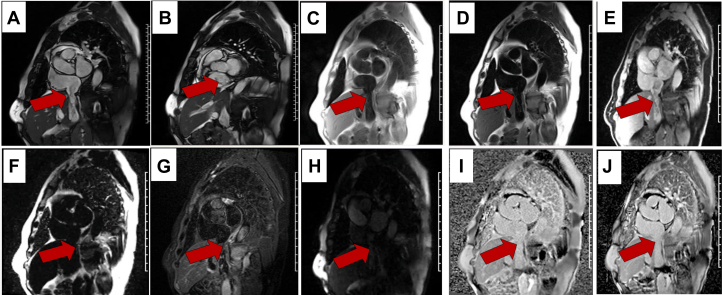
Cardiac MRI Findings Cardiac MRI demonstrating a lobulated, spongy intraluminal mass (red arrows) extending from the IVC into the RA, without evidence of myocardial invasion. (A and B) Cine steady-state free-precession (SSFP) images and (C) T1-weighted and (D) T2-weighted sequences show a mobile, T1- and T2-isointense mass arising from the IVC (red arrow in A) and projecting into the RA (red arrow in B), clearly separate from the atrial wall. Dixon (E) water-only and (F) fat-only images, along with the absence of signal suppression on (G) T2-weighted short tau inversion recovery (STIR), confirmed the lack of macroscopic fat within the lesion. (H) First-pass perfusion imaging demonstrated rapid, avid enhancement, indicating high vascularity and effectively excluding thrombus. Both (I) phase-sensitive inversion recovery (PSIR) LGE imaging and (J) long-TI imaging showed complete gadolinium enhancement of the IVC and RA mass, indicating the absence of fibrosis, necrosis, or myocardial infiltration and supporting tissue characterization consistent with tumor rather than bland thrombus. IVC = inferior vena cava; LGE = late gadolinium enhancement; MRI = magnetic resonance imaging; RA = right atrium.
